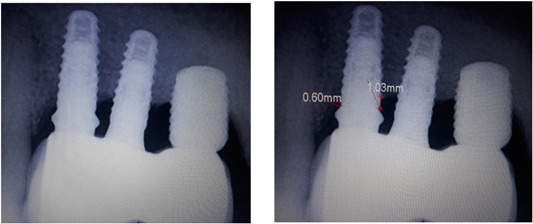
Radiographic assessment: The radiographic bone level is defined as the distance from the implant platform to the first implant/bone contact at the mesial and distal aspects of the implant.
